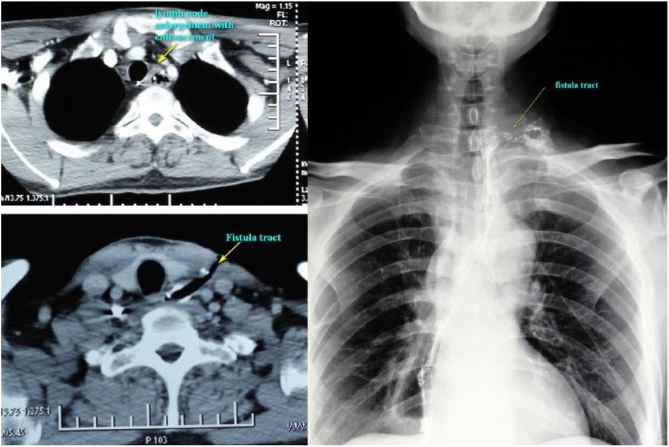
Enhanced MRI/CT scan and barium swallow exam demonstrated a fistulous tract from the anastomotic site to the lateral neck with enlarged lymph nodes found in level IV.
